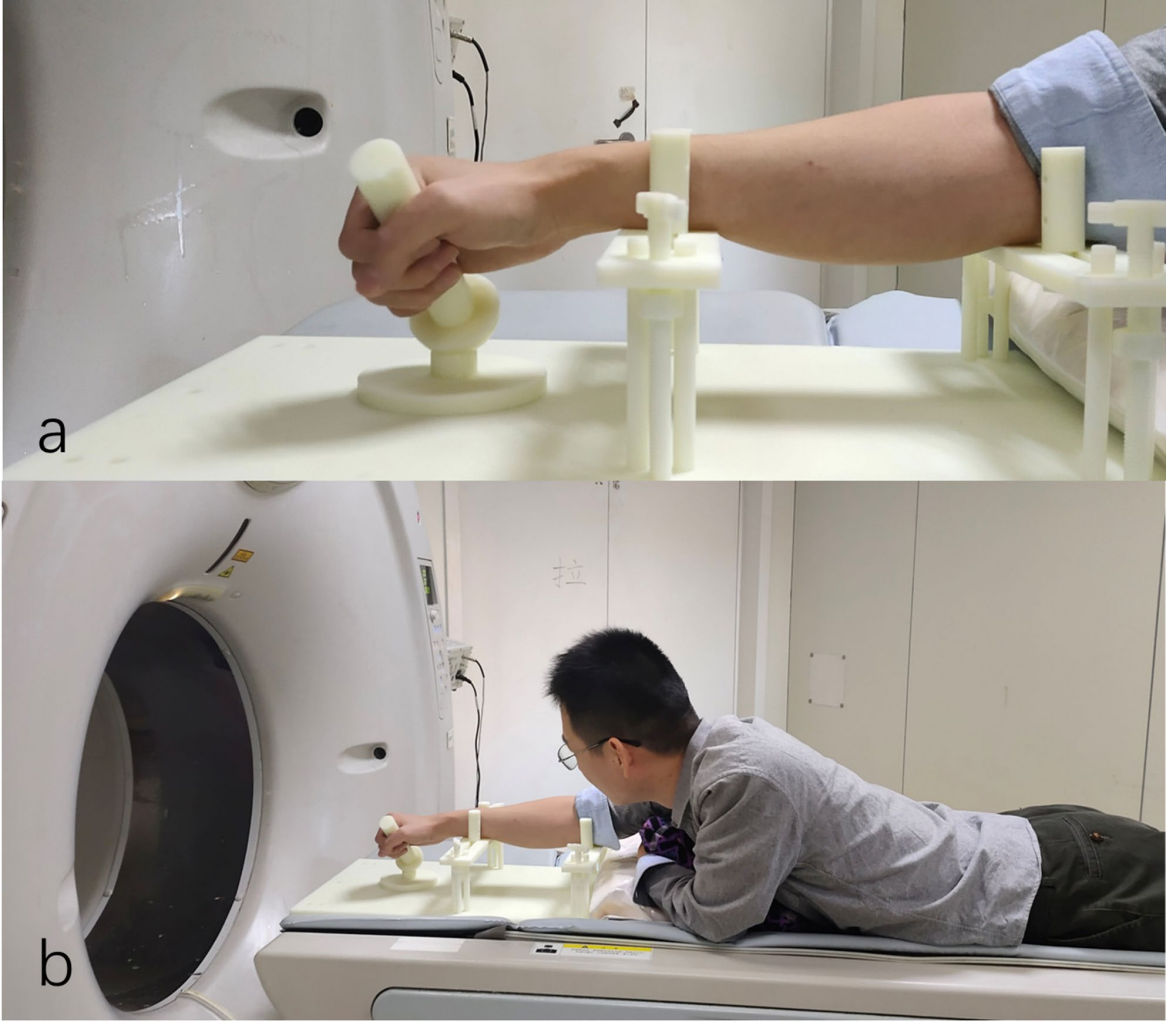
a Custom-made motion guidance apparatus used for standardizing hand and wrist kinematics. b Representative positioning of a subject during 4D CT acquisition
